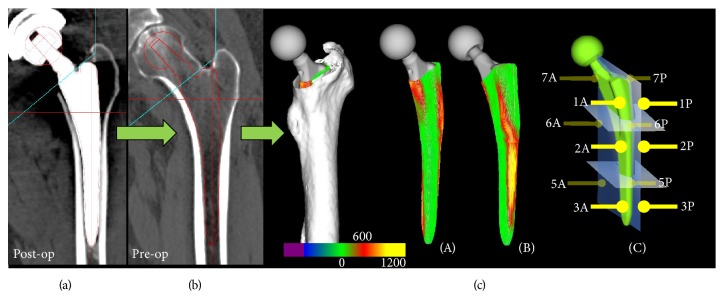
Virtual implant model generated from CAD data (a) and aligned and fitted to the contour of the real prosthetic stem postoperatively. The virtual model was aligned in the same position with a preoperative CT image (b). The contact pattern images were three-dimensionally visualized using a color map based on the CT values around the stem (c). The stem was defined as being in contact with the cortical bone if the CT value around the stem was ≥600 HU. Red color indicates 600 HU and yellow color indicates 1200 HU. (A) Anteromedial view. (B) Anterolateral view. (C) Modified Gruen zone classification; for example, zone 2A/zone 2P indicates the anterior/posterior portion of zone 2.
